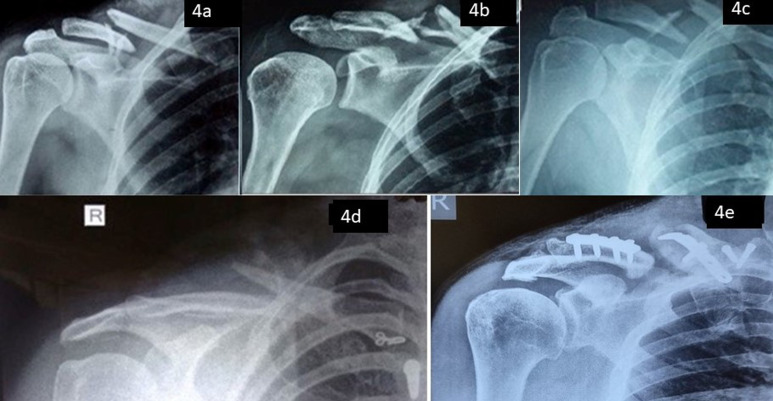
(a)–(c) Preoperative radiographs of fractures with non-union (4d) Nonunion after non-operative treatment (b) Nonunion after operative management.
